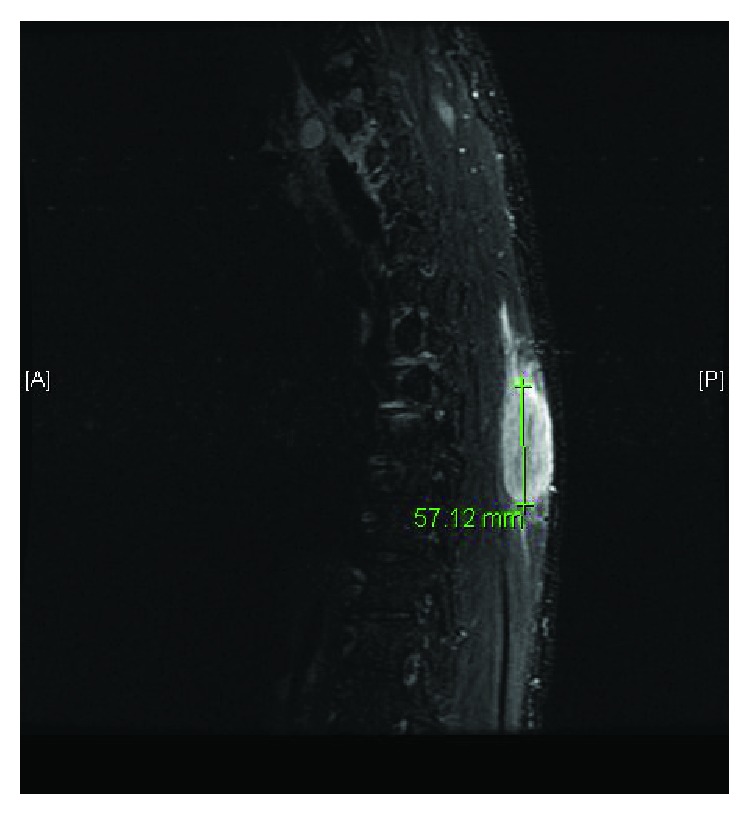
Intramuscular mass well demarcated with MRI imaging.
